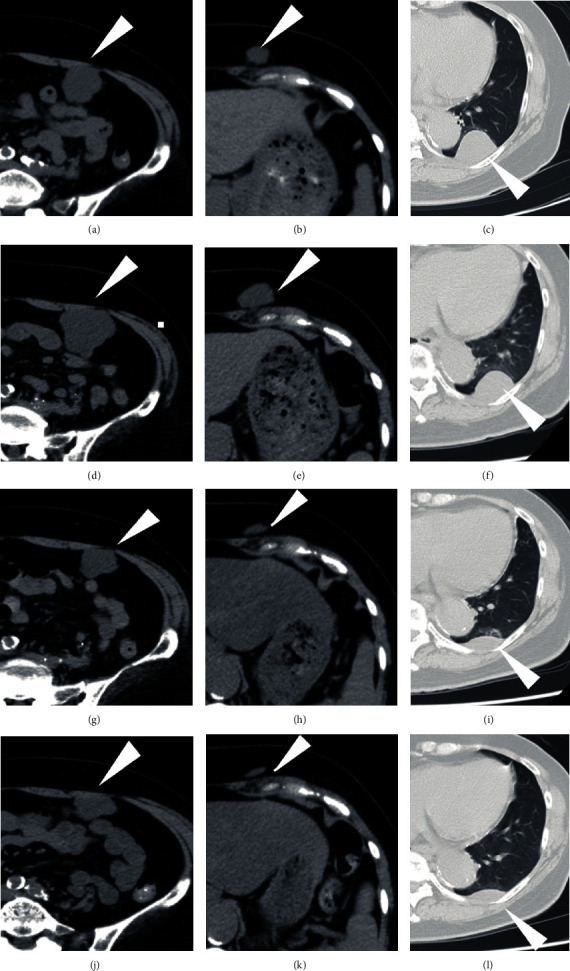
Response to chemotherapy in metastatic myxoid liposarcoma. Computed tomography (a–c) before and (d–f) after 3 cycles of trabectedin showing progressive disease. Partial response was obtained 5 months after treatment with eribulin (g–i). The tumors were under control for another 10 months (j–l).
